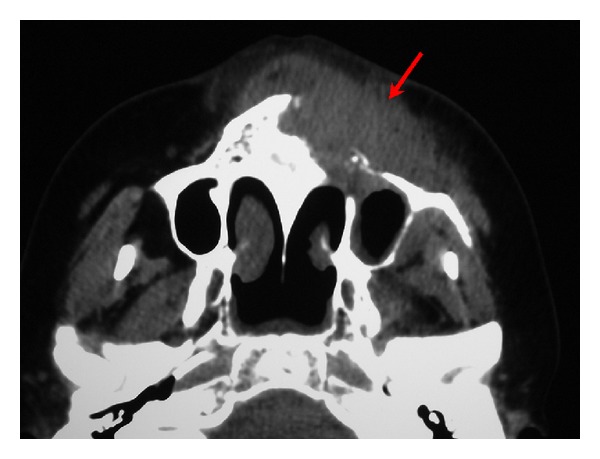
Axial CT image showing an infiltrative mass in the left maxillary region with destruction of the maxillary bone anteriorly wall, invading skin.
